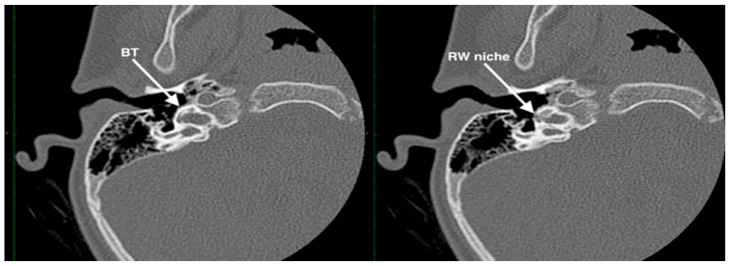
CT scan showing abnormal appearance of the promontory, with difficult interpretation of the middle ear landmarks and the basal turn intraoperatively—patient 1 (RW—round window; BT—basal turn of the cochlea).
